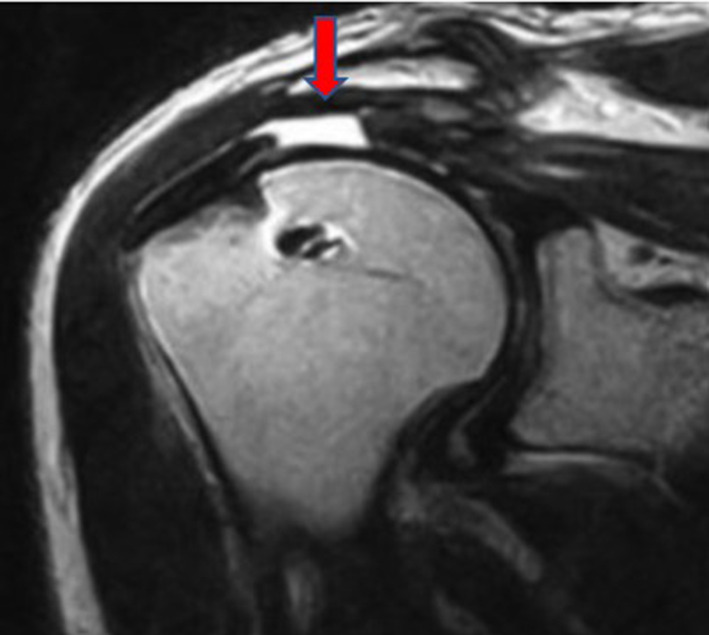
MRI of supraspinatus tear Sugaya type IV
